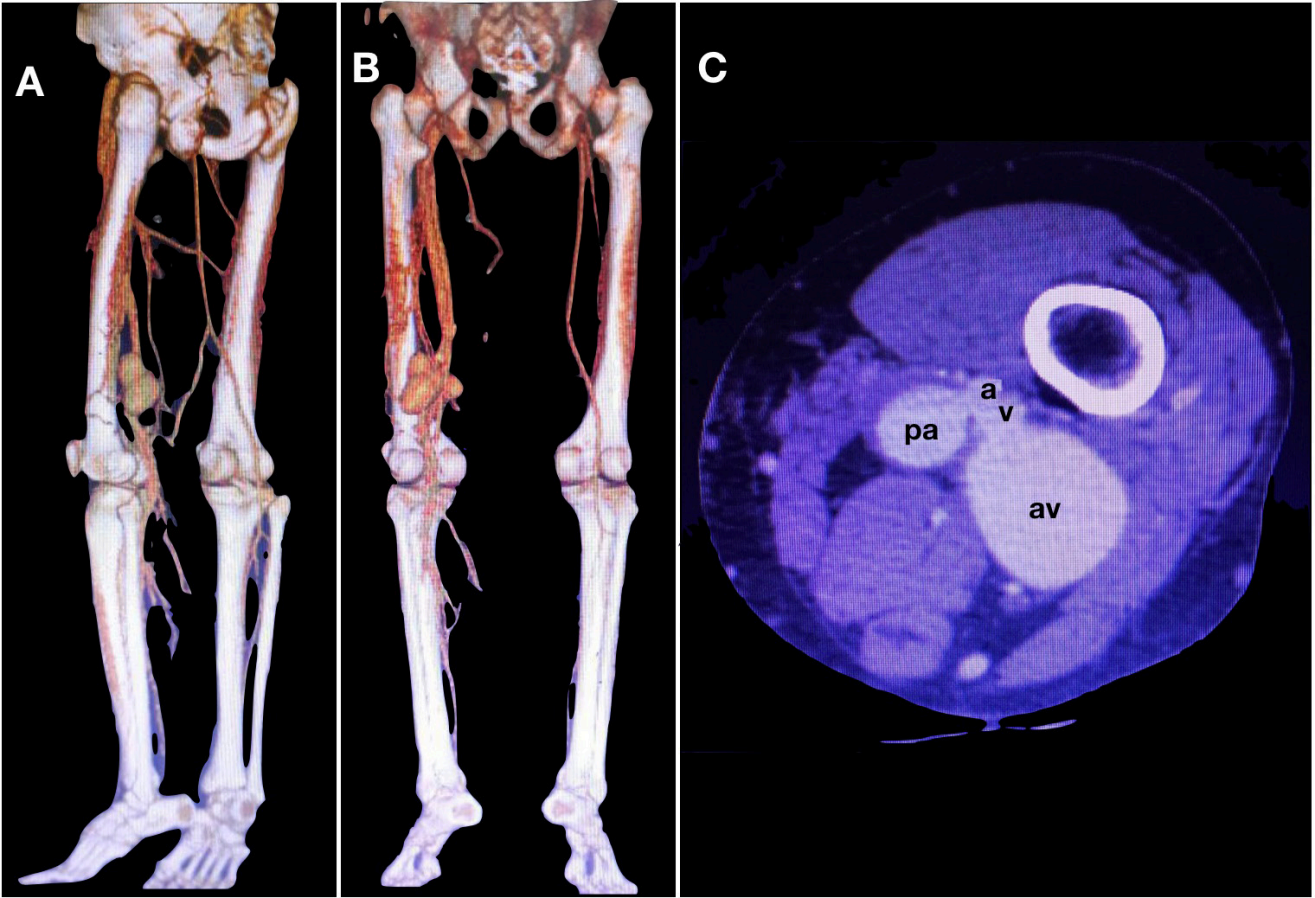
(A) and (B) Angiotomography reconstructions from different angles, showing simultaneous impregnation of the arterial system and the deep vein system in the left thigh by contrast and large dilatations of the popliteal vessels; (C) Axial computed tomography of the left lower limb; a: popliteal artery; pa: pseudoaneursym of the popliteal artery; v: popliteal vein; av: aneurysm of the popliteal vein.
